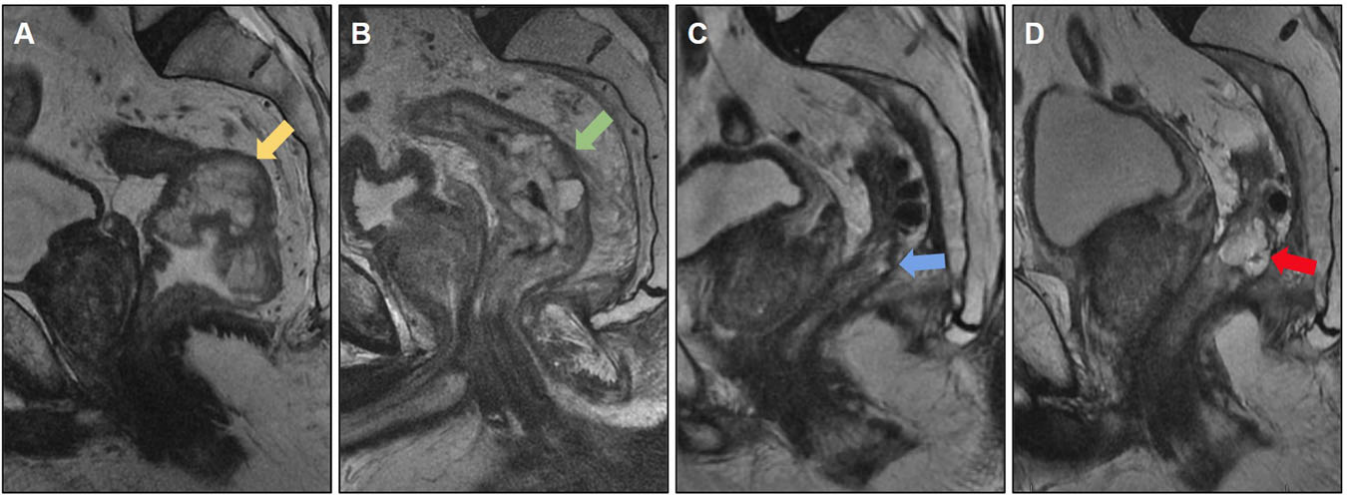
A Baseline MRI showing mucinous adenocarcinoma, mrT2N2 stage, negative mesorectal fascia (MRF), and negative extramural vascular invasion (EMVI) (yellow arrow). B MRI 8 weeks after neoadjuvant chemoradiotherapy (CRT) displaying poor response with tumor regression grade (TRG 5) (green arrow). C MRI 6 months after rectosigmoidectomy revealing incomplete mesorectal resection and insufficient distal margin (0.7 cm). The colorectal anastomosis exhibits a discrete area with a high T2 signal, casting doubt on postoperative changes or mucin (blue arrow). D MRI 1.5 years post-surgery demonstrating recurrence at the anastomosis site. Tissue growth with a high T2 signal was suggestive of recurrent tumor (red arrow). Subsequent surgical planning involved posterior abdominoperineal resection (APR)
